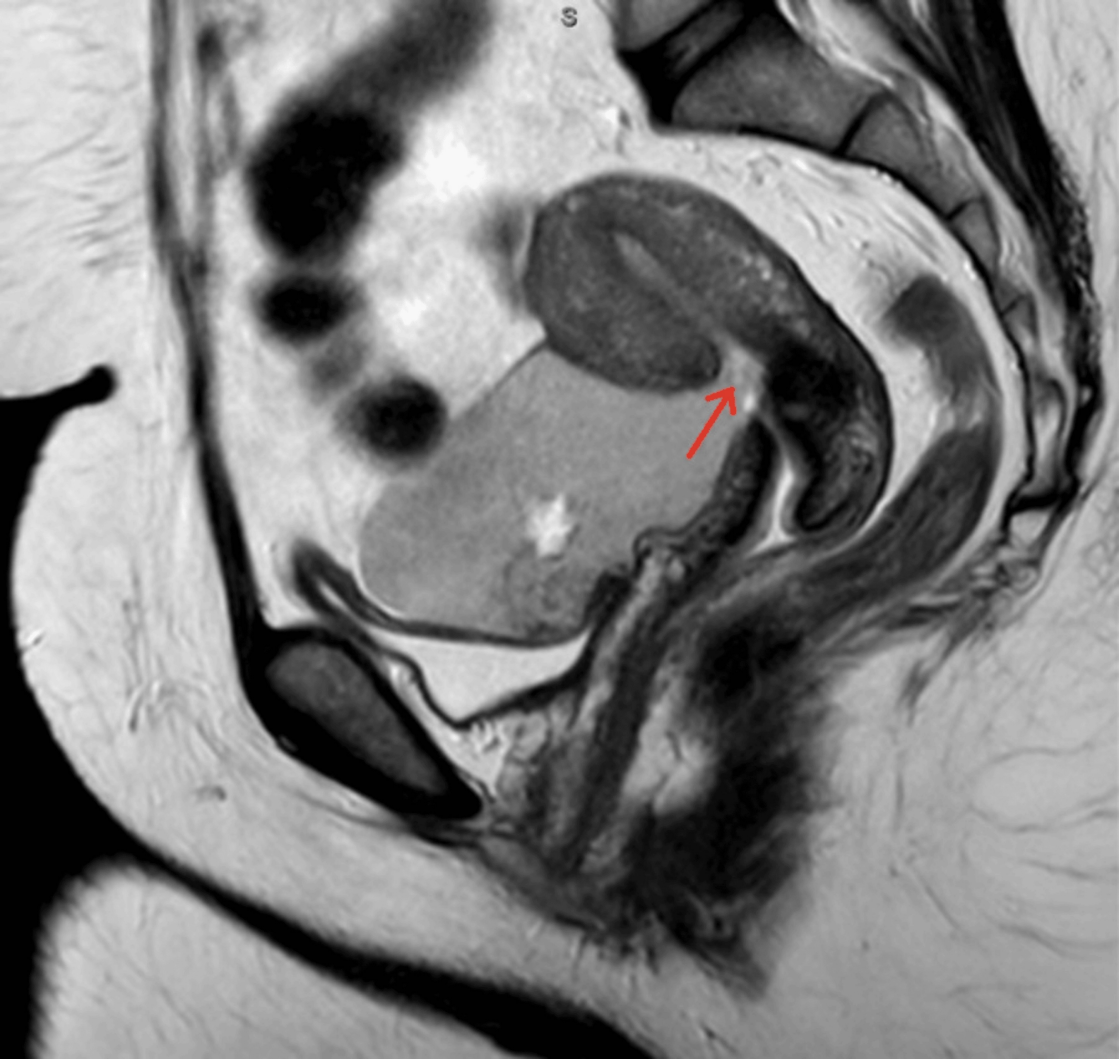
MRI demonstrating the large isthmocele
